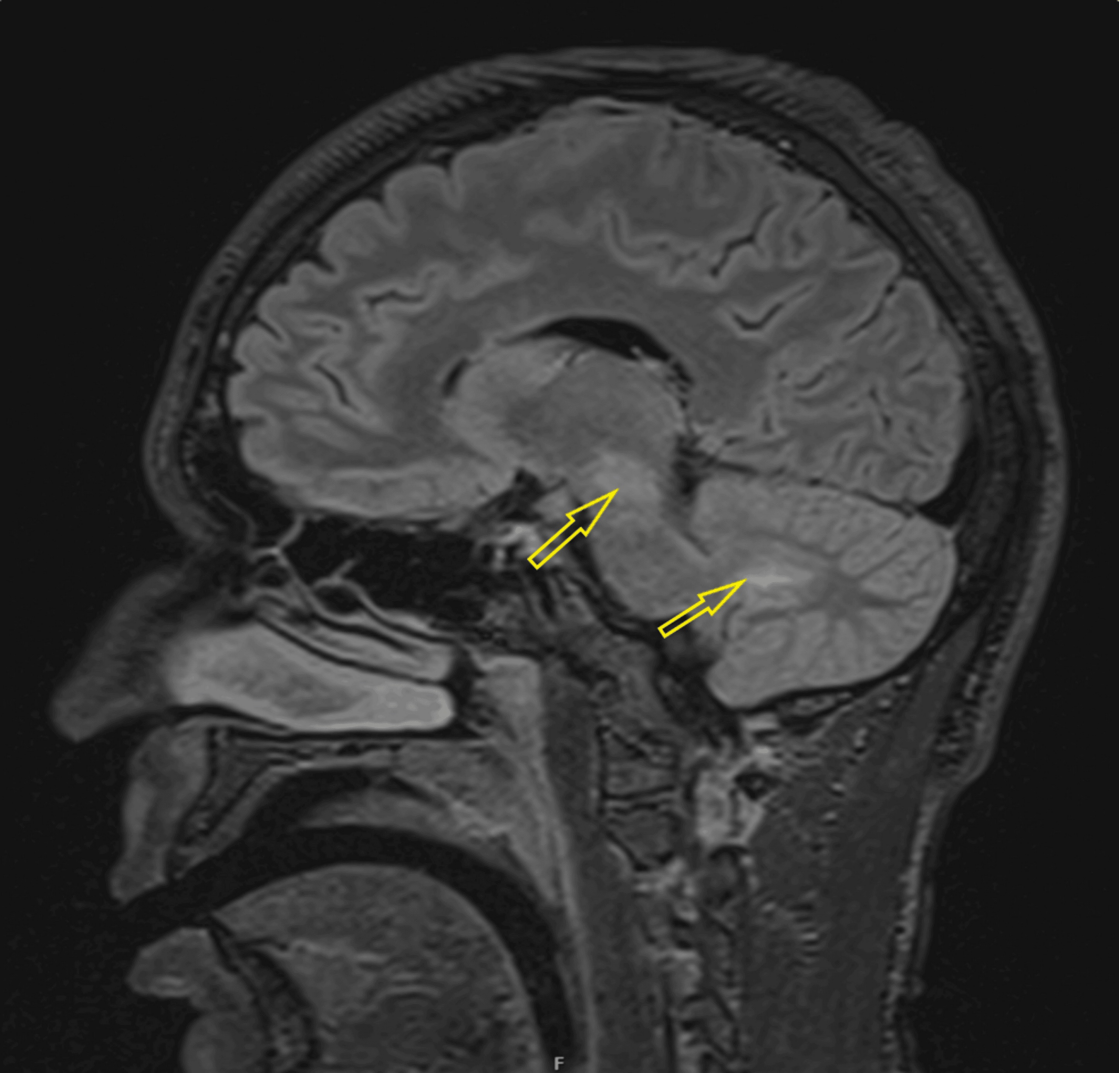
Sagittal fluid-attenuated inversion recovery (FLAIR) MRI demonstrating signal hyperintensity and edema in the dorsal midbrain and cerebellar white matter.
